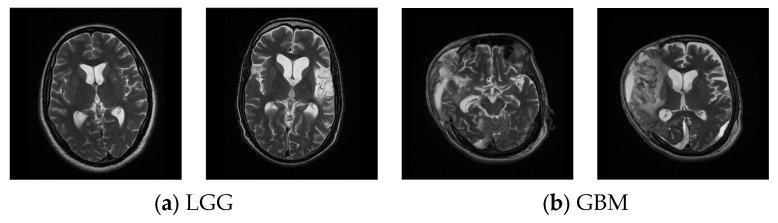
Clinically collected T2-modality MRI slices. (a) LGG, (b) GBM.
